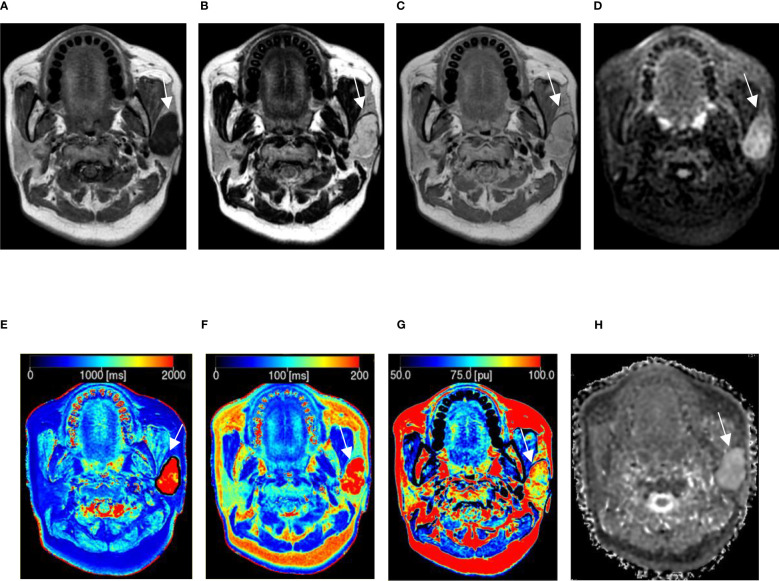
A 54-year-old woman with pleomorphic adenoma in the left parotid gland. (A–C) Proton density (PD), T1, and T2 images obtained from synthetic MRI. (D) Stimulus and fast spin echo diffusion-weighted imaging with periodically rotated overlapping parallel lines with enhanced reconstruction (b = 800 s/mm^2^). (E–G) Synthetic MRI-derived indicate that the mean T1, T2, and PD values measured by the two radiologists were 2227.50 ms, 265.17 ms, and 89.15 pu, respectively. (H) ADC map indicates that the mean apparent diffusion coefficient (ADC) value measured by the two radiologists was 2.40 × 10^-3^ mm^2^/s.
